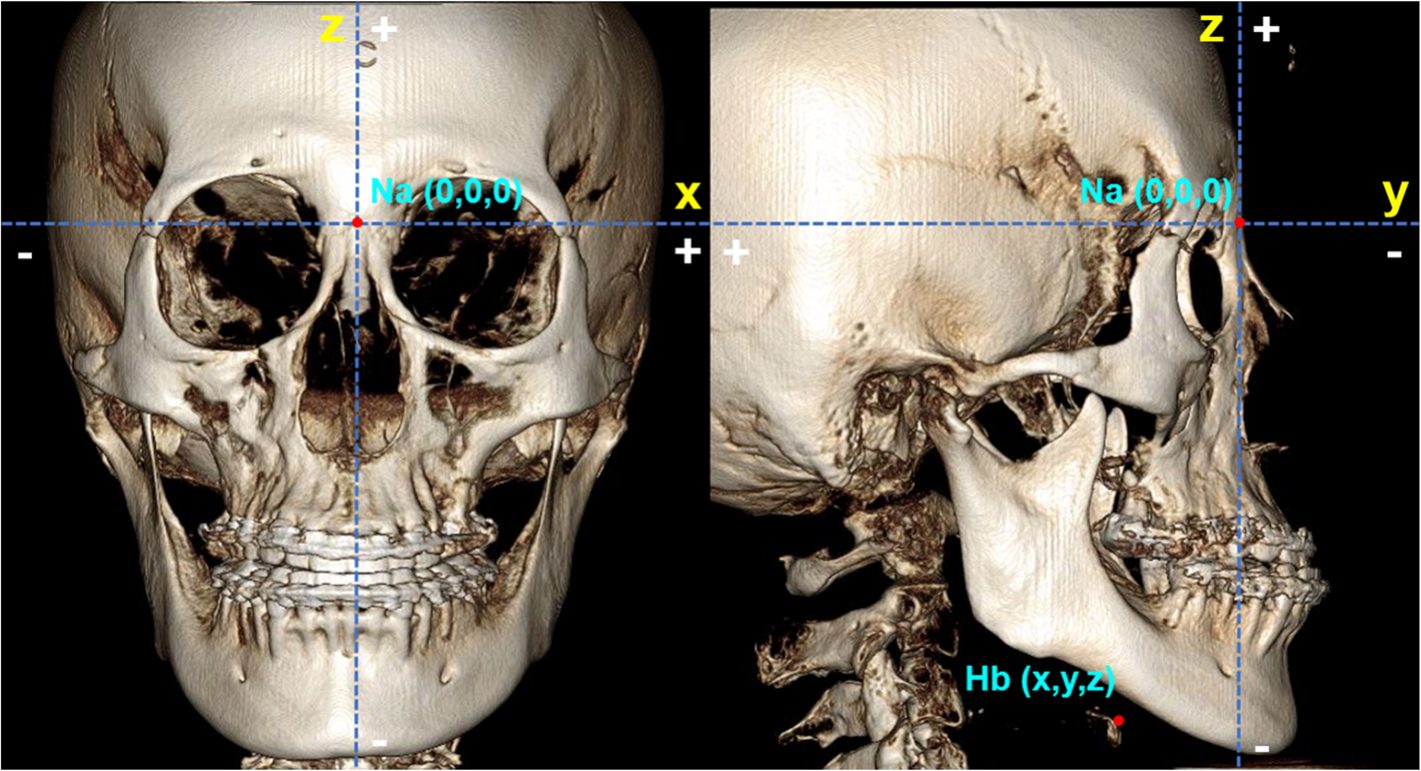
The plus and minus values along the three axes used in this study. The coordinates of the landmarks were assigned according to nasion (0, 0, 0). For the *x*-axis, landmarks on the left side are (+) values and the right side (−) values. For the *y*-axis, landmarks located posterior to the nasion are (+) values and anterior to the nasion (−) values. For the *z*-axis, landmarks located superior to the nasion are (+) values and inferior to the nasion (−) values. The coordinates of the Hb point are identified in T0 and T1 CBCT images
